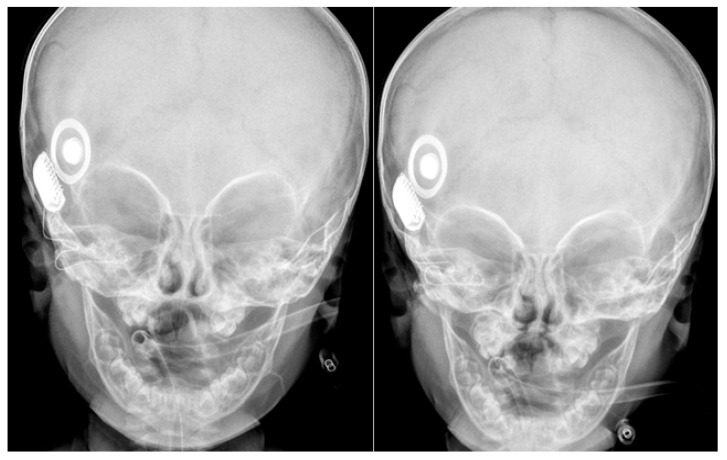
Intraoperative cochlear view X-ray in patient 5. A lateral wall electrode array misplaced inside the IAC (left). A second insertion led to correct placement (right).
